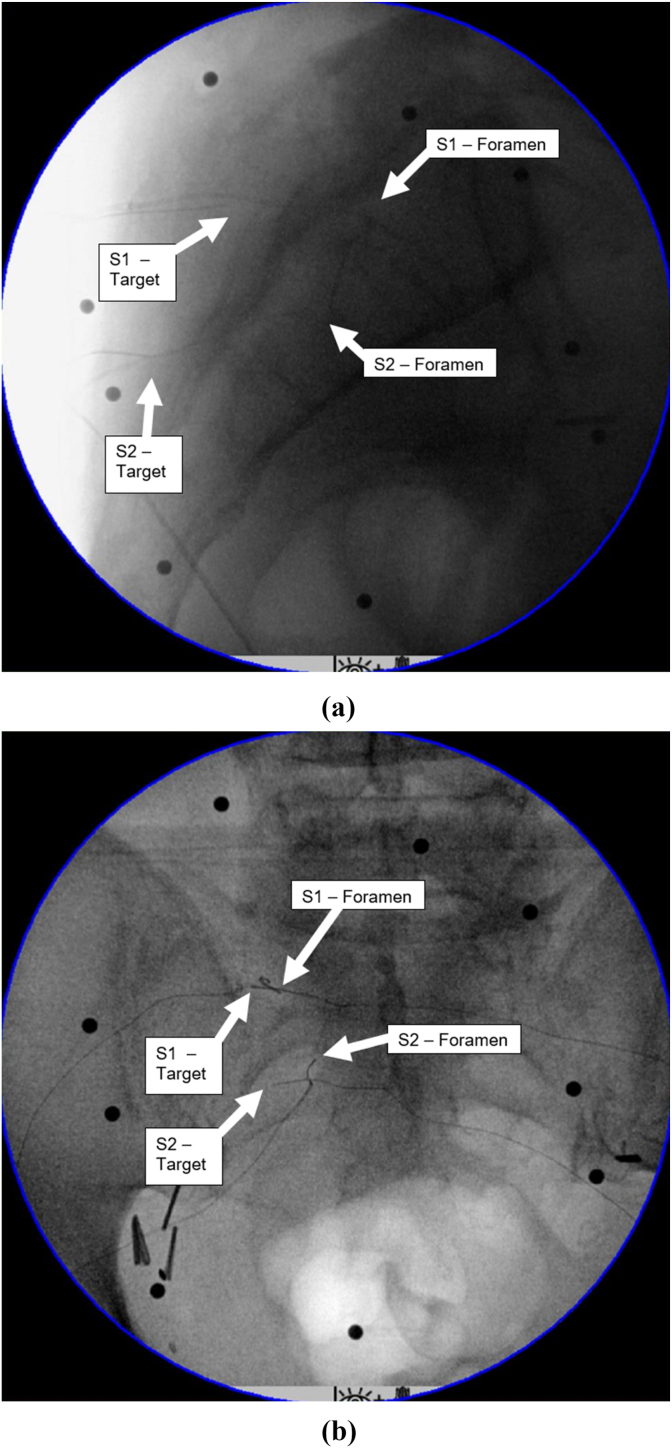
Labeled lateral (A) and posterior-to-anterior (B) x-rays of thermocouples post-implantation.
